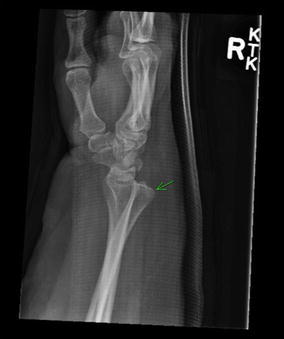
Lateral radiographs of the *right wrist* demonstrating distal radioulnar joint disruption and dorsal ulnar dislocation (*green arrow*)
